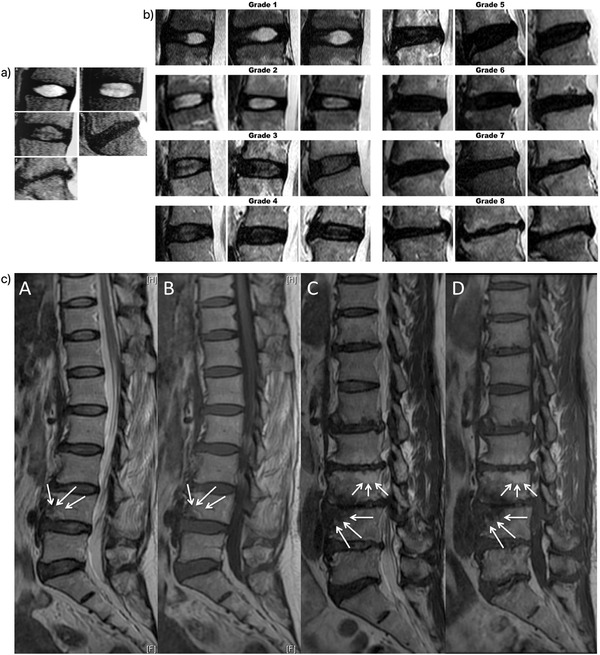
Classification system for IVD degeneration based on MRI pathological features. a) *Pfirrmann* grading system for the assessment of lumbar disc degeneration. Reproduced with permission.^[^
